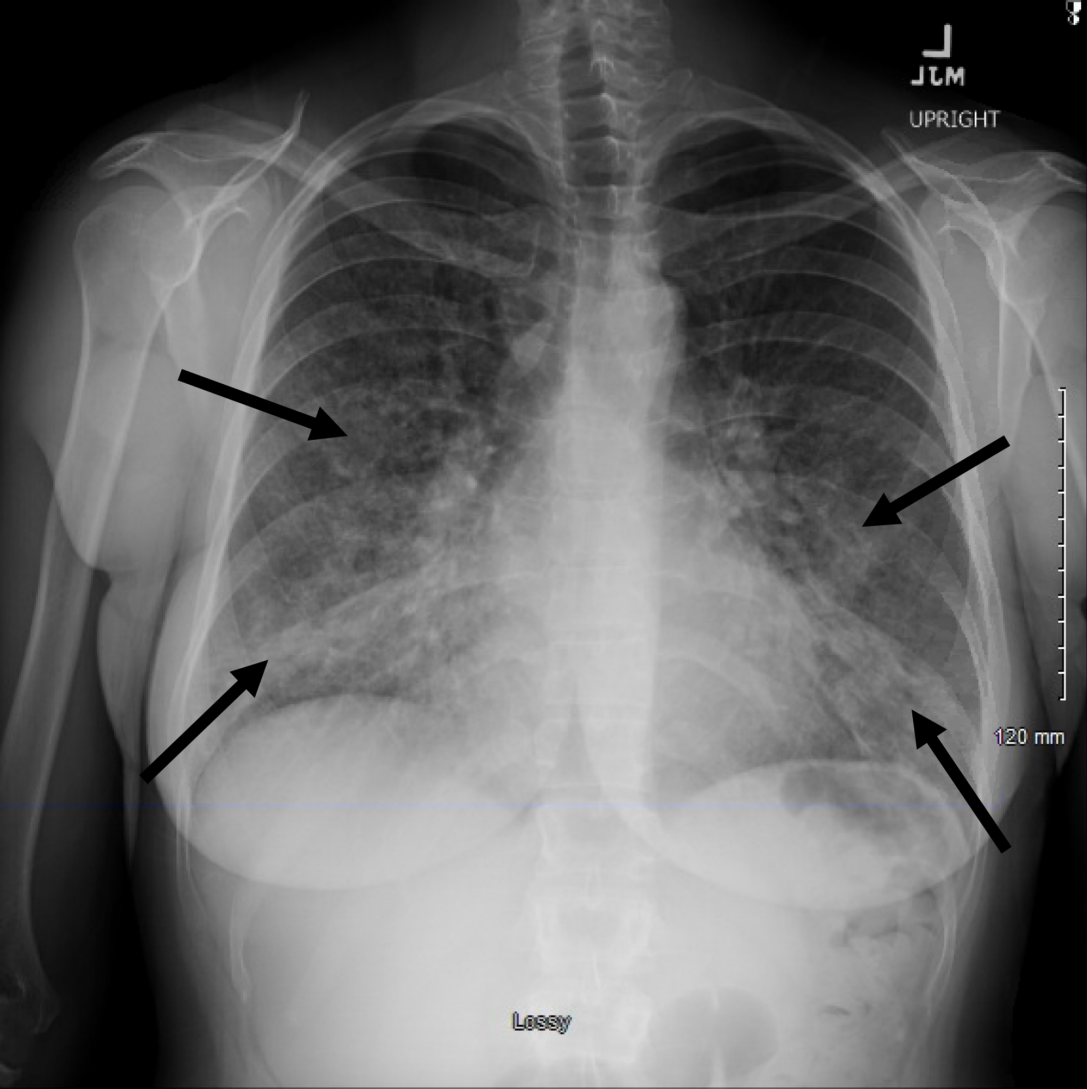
Chest X-ray (PA view) demonstrating diffuse bilateral lung infiltrates (indicated by arrows). PA, posteroanterior
